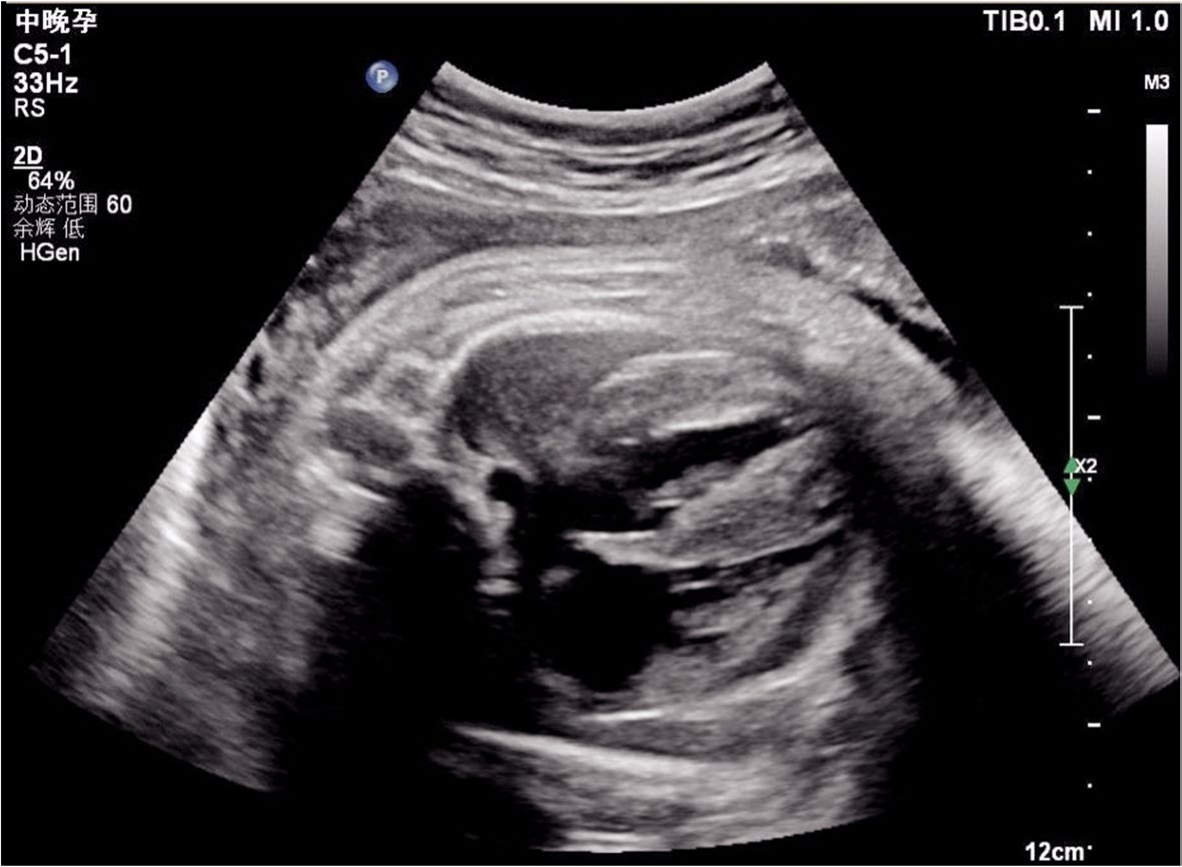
Echocardiogram demonstrating ventricular hypertrophy in utero
